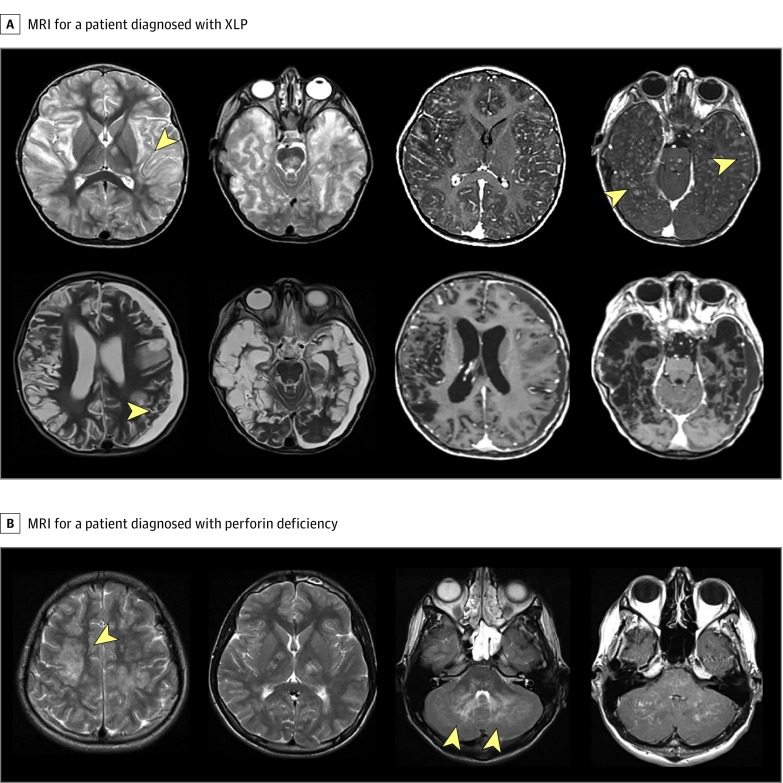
Neuroimaging Studies for 2 Patients Diagnosed With Primary Hemophagocytic Lymphohistiocytosis Following Sequencing on Neuroinflammation Gene Panel A, Magnetic resonance imaging (MRI) axial T2-weighted images from a patient diagnosed with X-linked lymphoproliferative (XLP) syndrome type 1 due to heterozygous c.163C>T variant in the SH2 domain containing 1A (SH2D1A) gene. In the top row, the 2 images to the left show extensive involvement of the subcortical white matter (arrowhead) affecting both cerebral hemispheres, thalami, and brainstem. To the right, 2 postgadolinium contrast images show extensive punctate enhancement (arrowheads) in the subcortical areas. In the bottom row, the 2 images on the left show follow-up imaging 7 months after initial presentation with marked brain atrophy with leukomalacia and a left-sided subdural collection (arrowhead). B, Brain MRI for a patient with perforin deficiency due to c.731T>G and c.694C>T variants in perforin gene. Signal changes are noted in both cerebral hemispheres, also affecting the capsular regions. Arrowheads indicate cerebellar signal abnormality.
